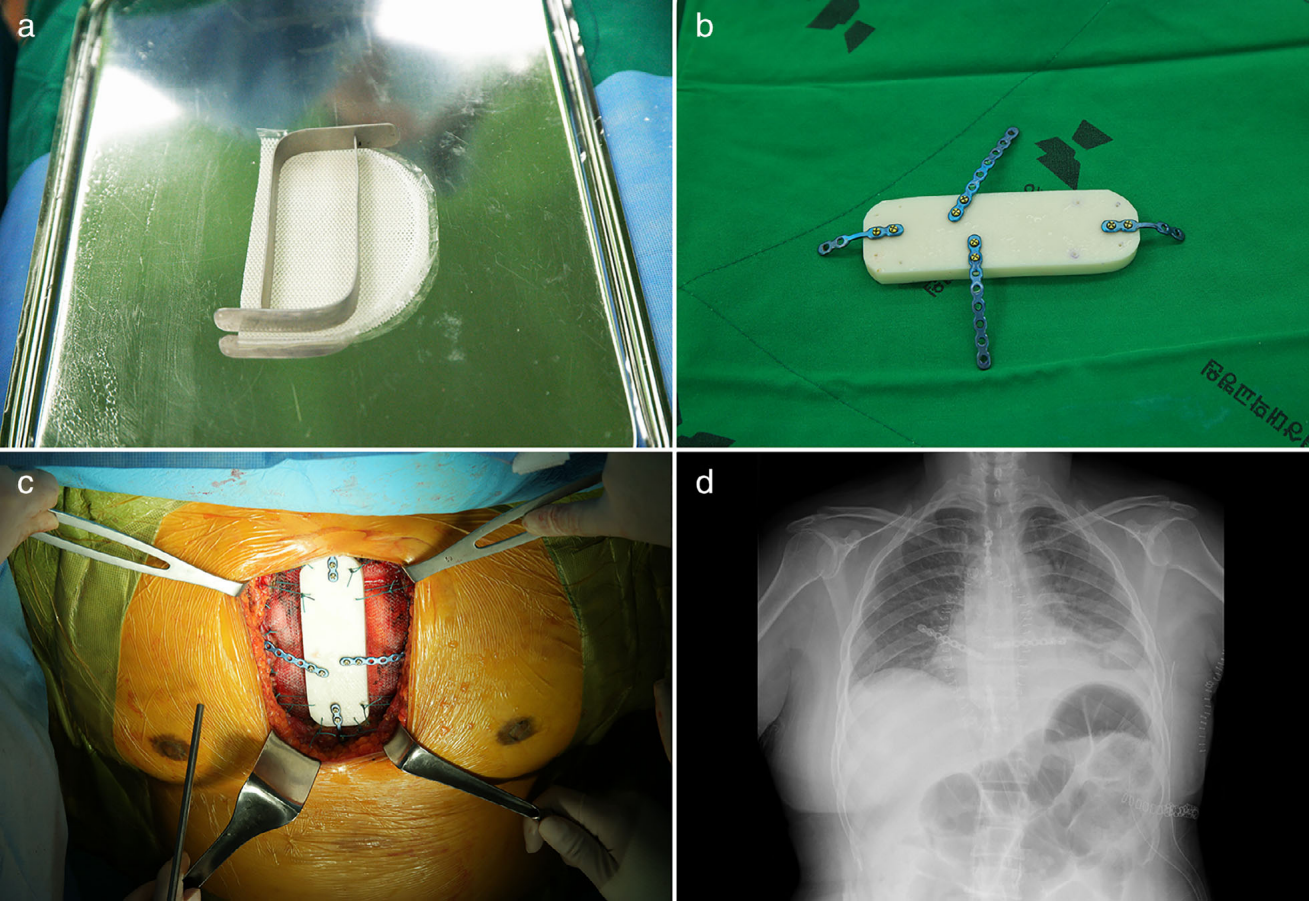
(a) The mesh was initially placed on the Mayo stand, and a malleable spatula used to mold the bone cement block. (b) After preparing the bone cement, a titanium plate was fixed to the bone cement block, initially to fit the rib structure, and thereafter to fit the remaining sternal structure. (c) The neosternum was placed after removal of the sternum. It was fixed to the ribs and the remaining sternum. Ribs 2 and 4 were fixed using 1–0 Ethibond suture. (d) Chest radiograph obtained three days postoperatively showed the neosternum constructed using a bone cement block.
